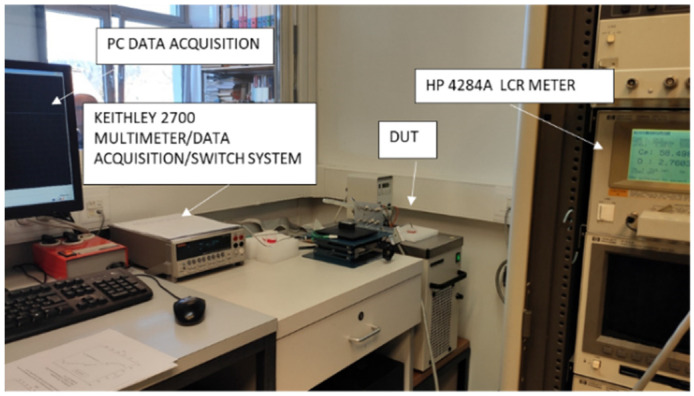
Measurement setup for CT sensor characterization by lab instruments. DUT is CT sensor inserted into Eppendorf tube and dipped into thermostated bath.
